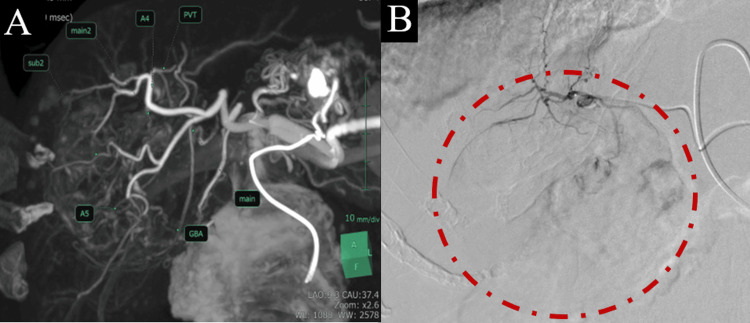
TACE performed on the day of admission for ruptured HCC A: Confirmation of the arterial anatomy with 3D CT angiography. B: Embolization of the right hepatic artery responsible for the ruptured HCC (red circle) CT: computed tomography; HCC: hepatocellular carcinoma; TACE: transcatheter arterial chemoembolization
